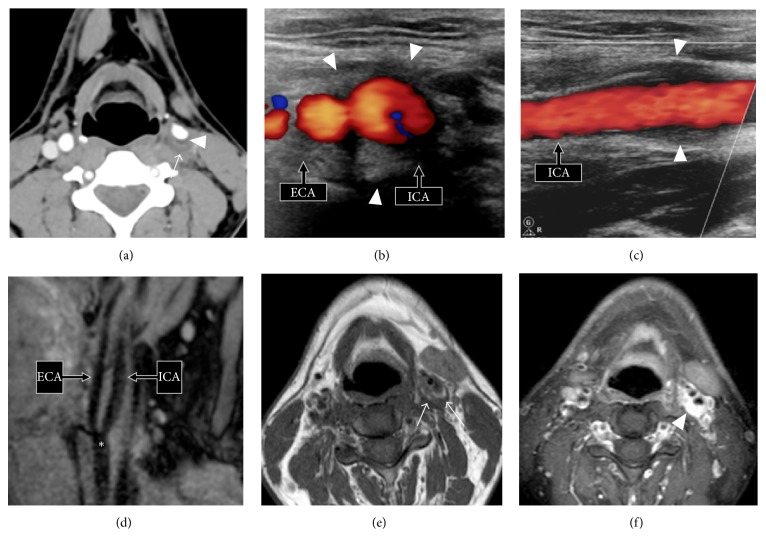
(a) Axial contrast-enhanced CT scan (soft tissue window) shows poorly delineated thickening of the left carotid bulb wall* (arrow)*. Note obliteration of the pericarotid fatty tissue. Minimal vessel narrowing (<20%) due to fibrolipid plaque* (arrowhead)*. (b, c) Axial and longitudinal views of Doppler US examination show fusiform eccentric wall thickening of the left proximal internal carotid artery (ICA) and external carotid artery (ECA) isoechoic to muscle* (arrowheads)*. (d) Sagittal multiplanar reformatted (MPR) image from a 3D volume turbo spin echo acquisition centered on the left carotid bulb* (asterisk)* shows no evidence of spontaneously T1 hyperintense parietal hematoma, thus ruling out carotid artery dissection. No aneurysm is seen. (e) Axial T1-weighted MR image reveals a hypointense circumferential lesion involving the left carotid bulb and pericarotid fatty tissue* (arrows)*. (f) Intense enhancement* (arrowhead)* of the mass-like soft tissue lesion on the axial postcontrast fat-saturated T1-weighted MR image. Note that perivascular enhancement and vessel wall enhancement can be hardly differentiated from one another. ICA: internal carotid artery and ECA: external carotid artery.
